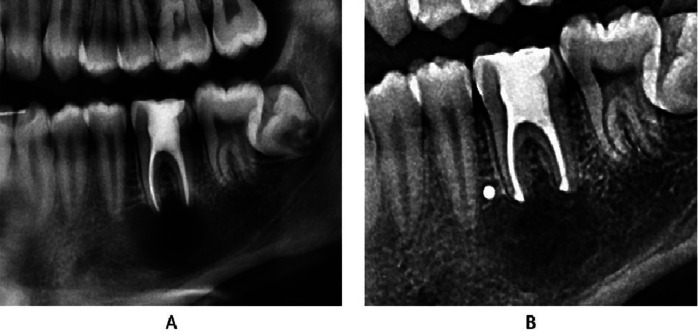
Orthopantomography images. A: Preoperative orthopantomography. Apical radiolucent zone of first mandibular molar was detected. B: Control orthopantomography 6 months after surgery. Bone regeneration can be detected.
